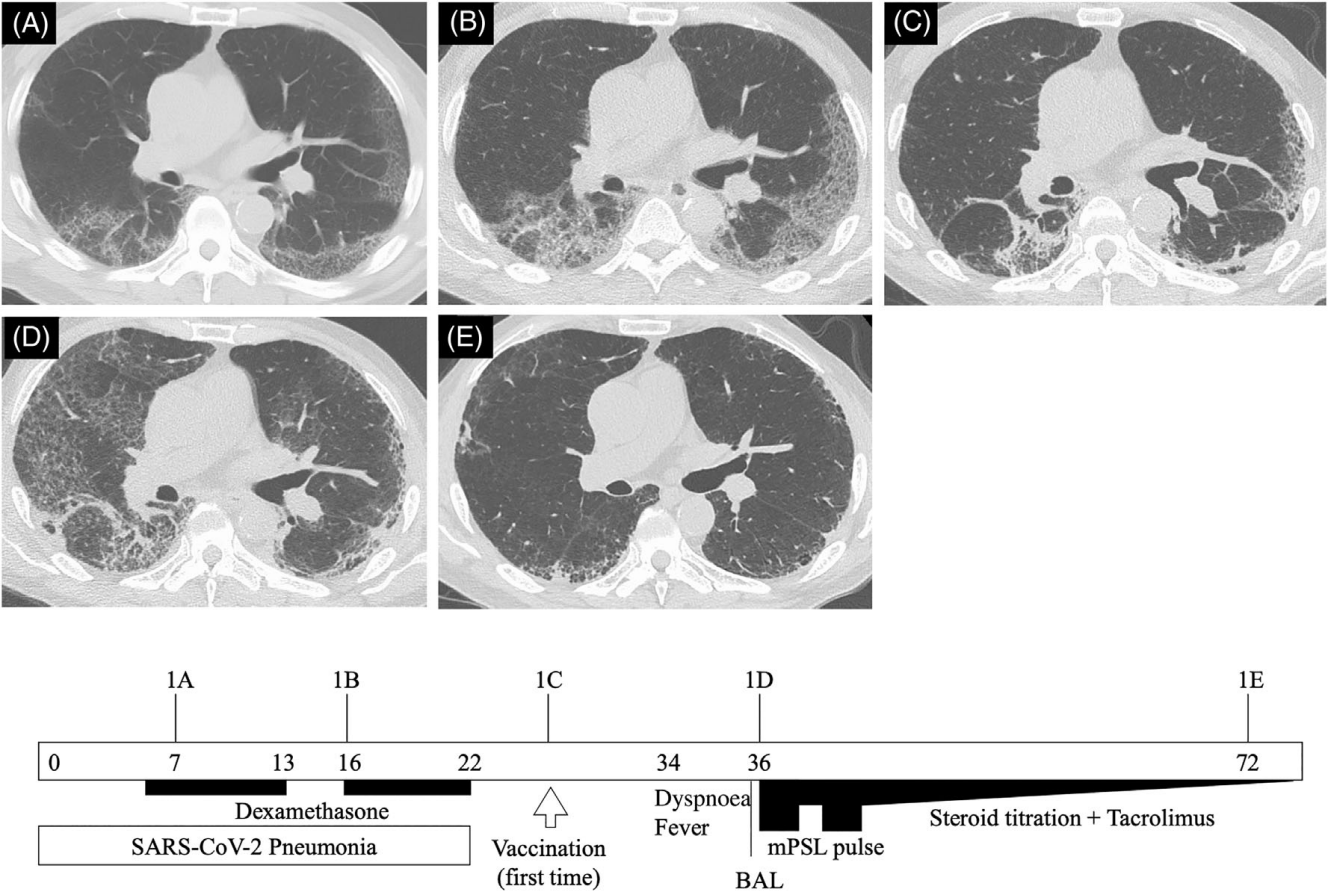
Simple clinical course of Case 1. Each HRCT corresponds to the figure numbers in the main text. Arabic numerals in the timeline square indicate the number of days since the onset of COVID‐19. BAL, bronchoalveolar lavage; COVID‐19, coronavirus disease 2019; HRCT, high‐resolution computed tomography; mPSL, methylprednisolone; SARS‐CoV‐2, severe acute respiratory syndrome coronavirus 2
